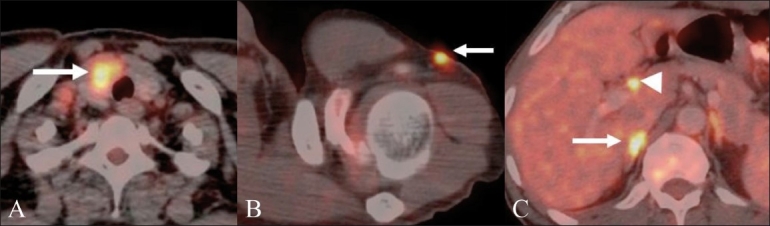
Restaging FDG PET / CT studies performed on three different patients of treated CRC for suspicion of disease recurrence show unusual metastatic sites; in the right lobe of the thyroid gland (arrow in A), in the subcutaneous region of the left upper arm (arrow in B), metastatic portal adenopathy (arrowhead in c), and a right adrenal metastasis (arrow in C)
